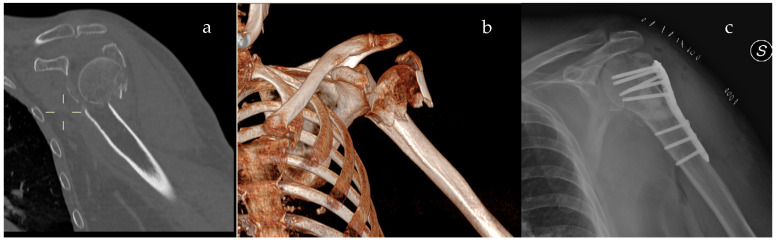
(a) Radiographic images and (b) 3D CT reconstruction of fracture with valgus breakdown and bone gap. On the right, (c) post-operative evaluation.
